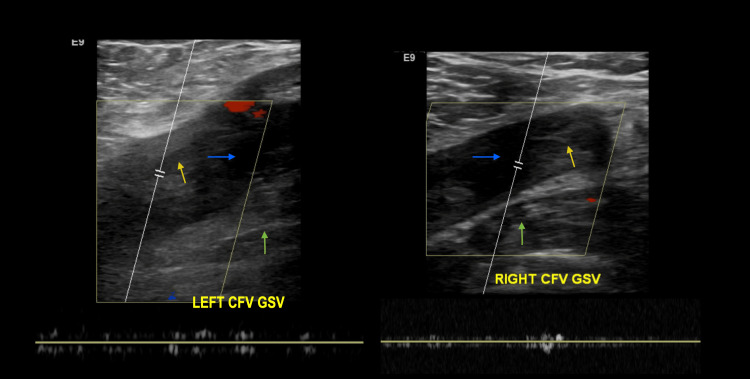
Ultrasound of the bilateral lower extremities showing occlusive echogenic filling defects (yellow arrow) in bilateral common femoral veins with no flow, consistent with DVT. CFV: common femoral vein (blue arrow), GSV: greater saphenous vein (green arrow), DVT: deep vein thrombosis
